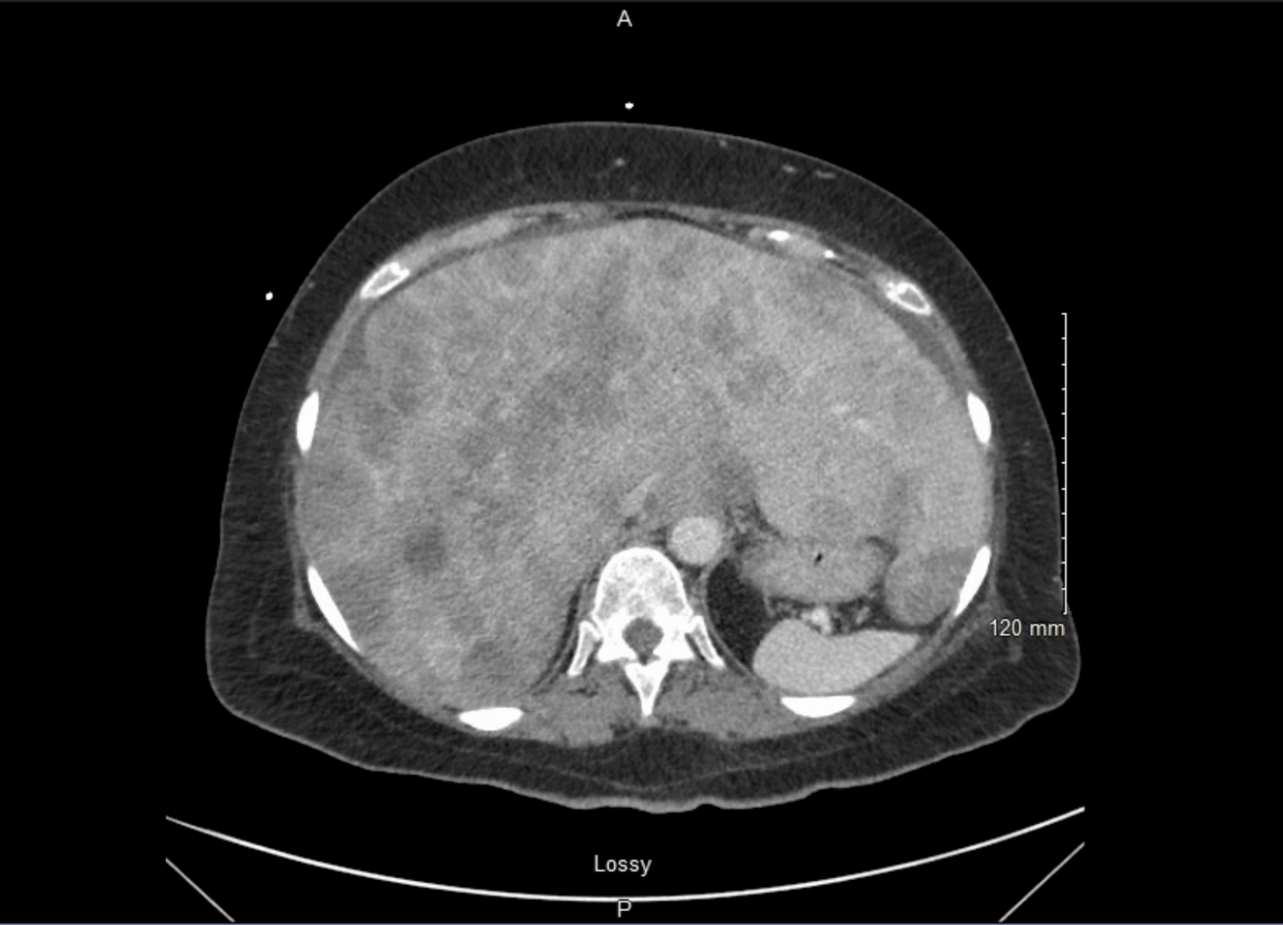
Axial view of CT abdomen and pelvis showing hepatic densities consistent with the patient’s history of stage IV cervical cancer with hepatic metastases and small-volume ascites.
